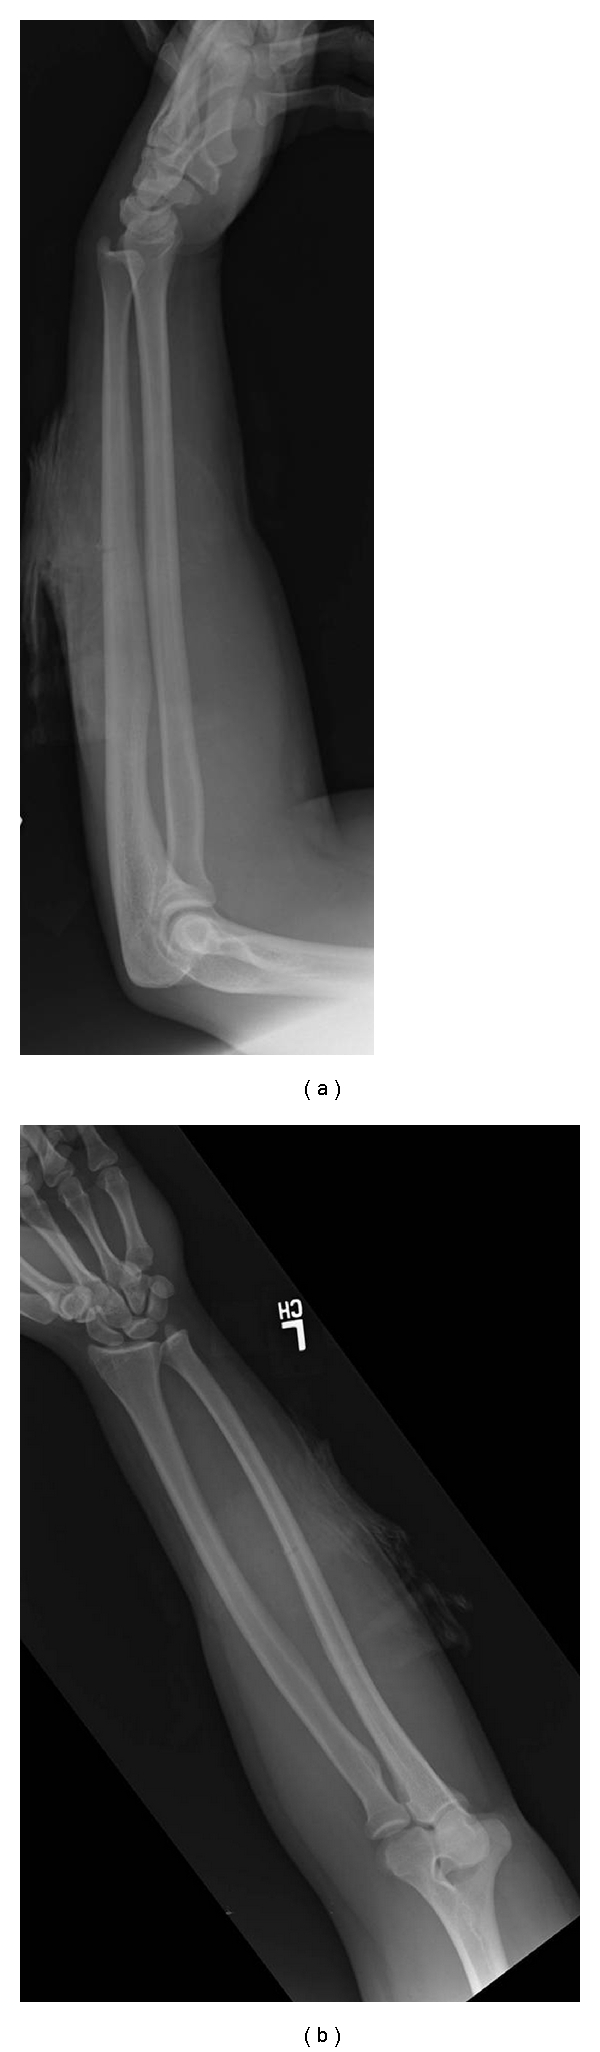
Preoperative (a) anteroposterior and (b) lateral forearm radiographs of 17-year-old right hand dominant male who was struck with a machete on his left dorsal forearm.
